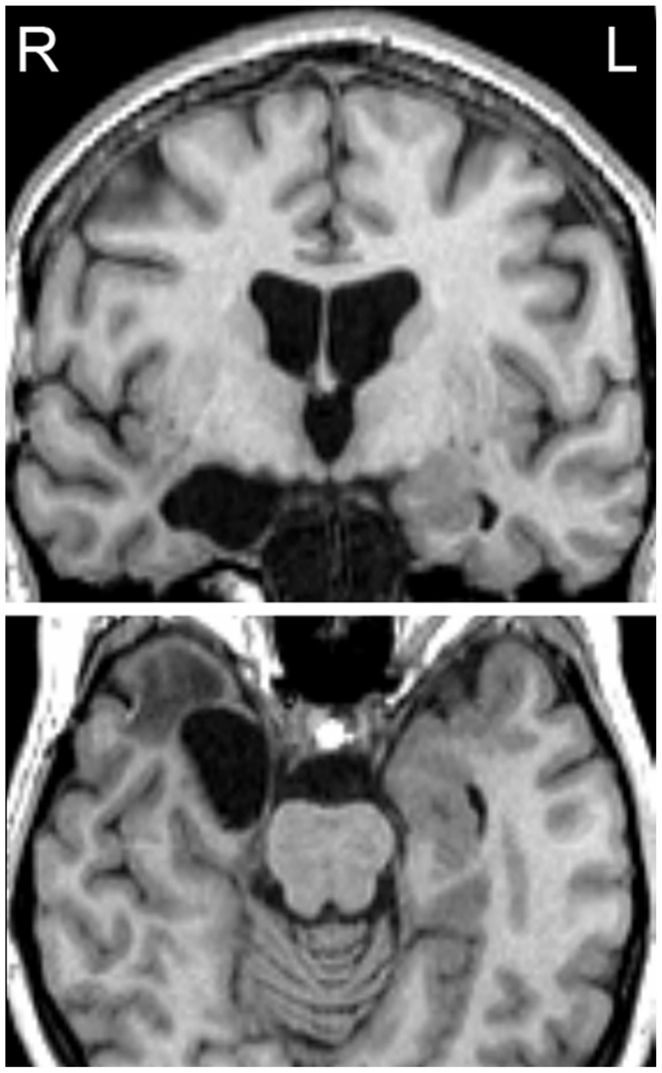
Example lesion, patient H.N. Top: coronal MRI section perpendicular to the line connecting the anterior and posterior commissures (AC-PC line), at the level of amygdala, hippocampal head, rostral entorhinal cortex, rostral perirhinal cortex and infero-temporal cortex. Bottom: Axial MRI section parallel below the AC-PC line, at the level of amygdala, rostral hippocampus, entorhinal cortex, perirhinal cortex and infero-temporal cortex. Note damage to rostral hippocampus and adjacent MTL structures on the right.
